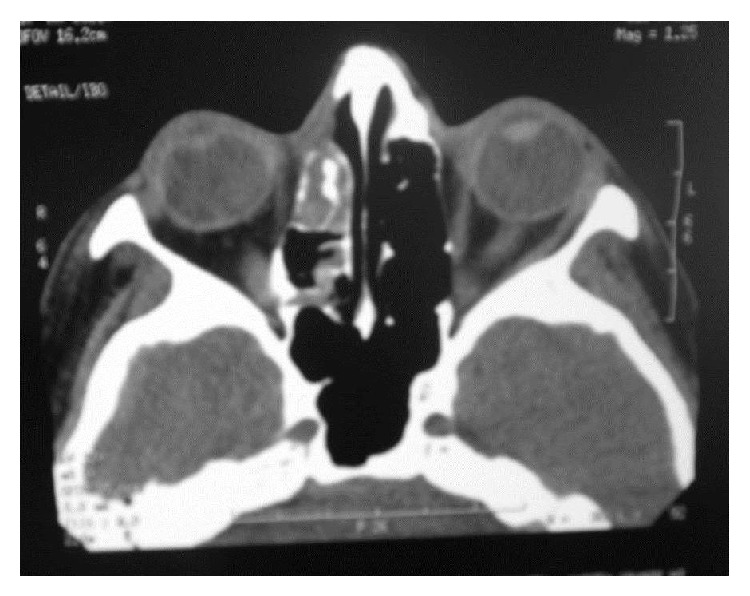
CT section of the region.
